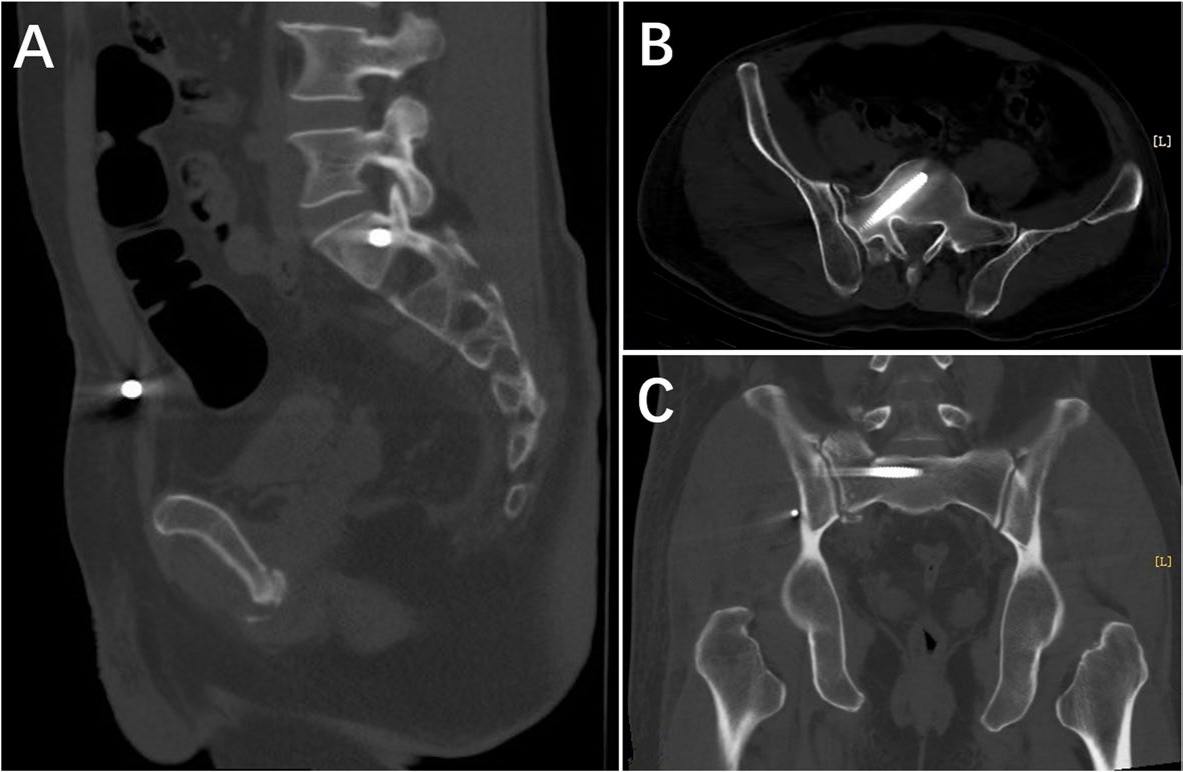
A: Postoperative day 3 sagittal CT showing the iliosacral screw position; B: transverse section showing sacral-fracture reduction; C: coronal section showing fracture reduction and the iliosacral screw position
